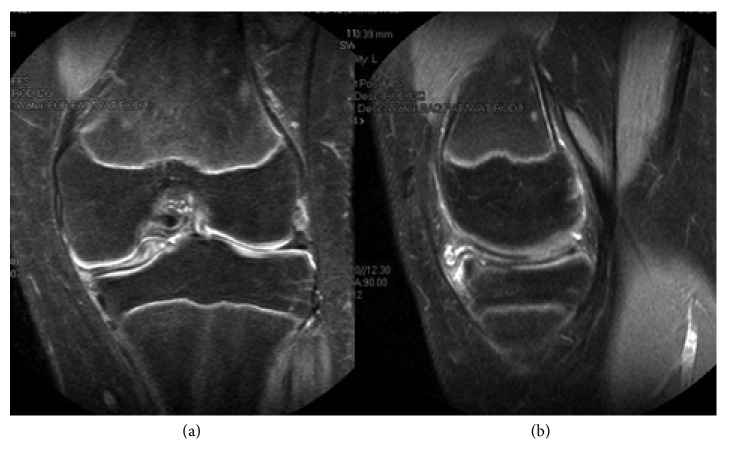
MRI of the left knee. (a) Coronal view showing the upper side of the medial meniscus folded in the intercondylar notch. (b) Sagittal view demonstrating horizontal and vertical peripheral tear.
